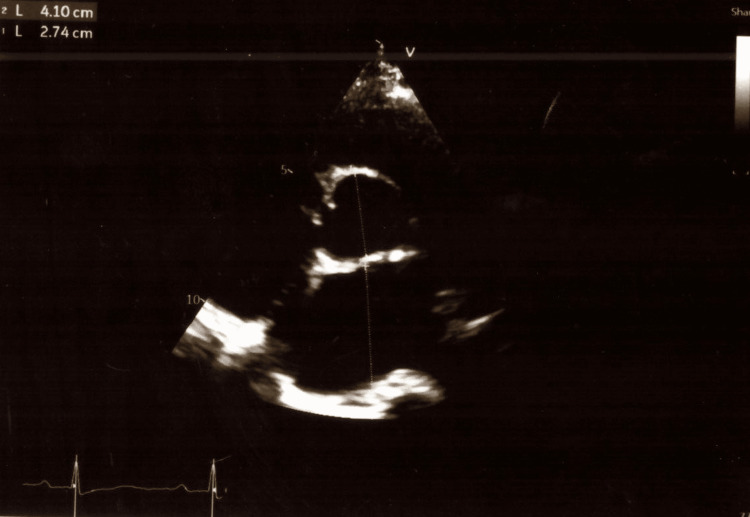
Two-dimensional echocardiogram from the patient with mitral valve stenosis
